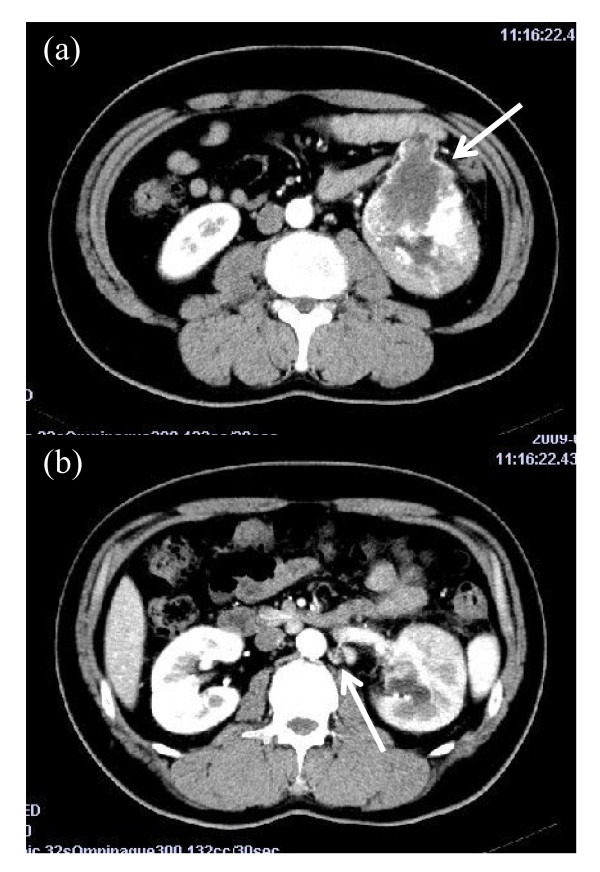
Abdominal enhanced computed tomography scan at diagnosis. (a) A tumor of the left kidney measuring 7.2 × 6.5cm (arrow); (b) lymph node swelling in the renal hilar region (arrow).
